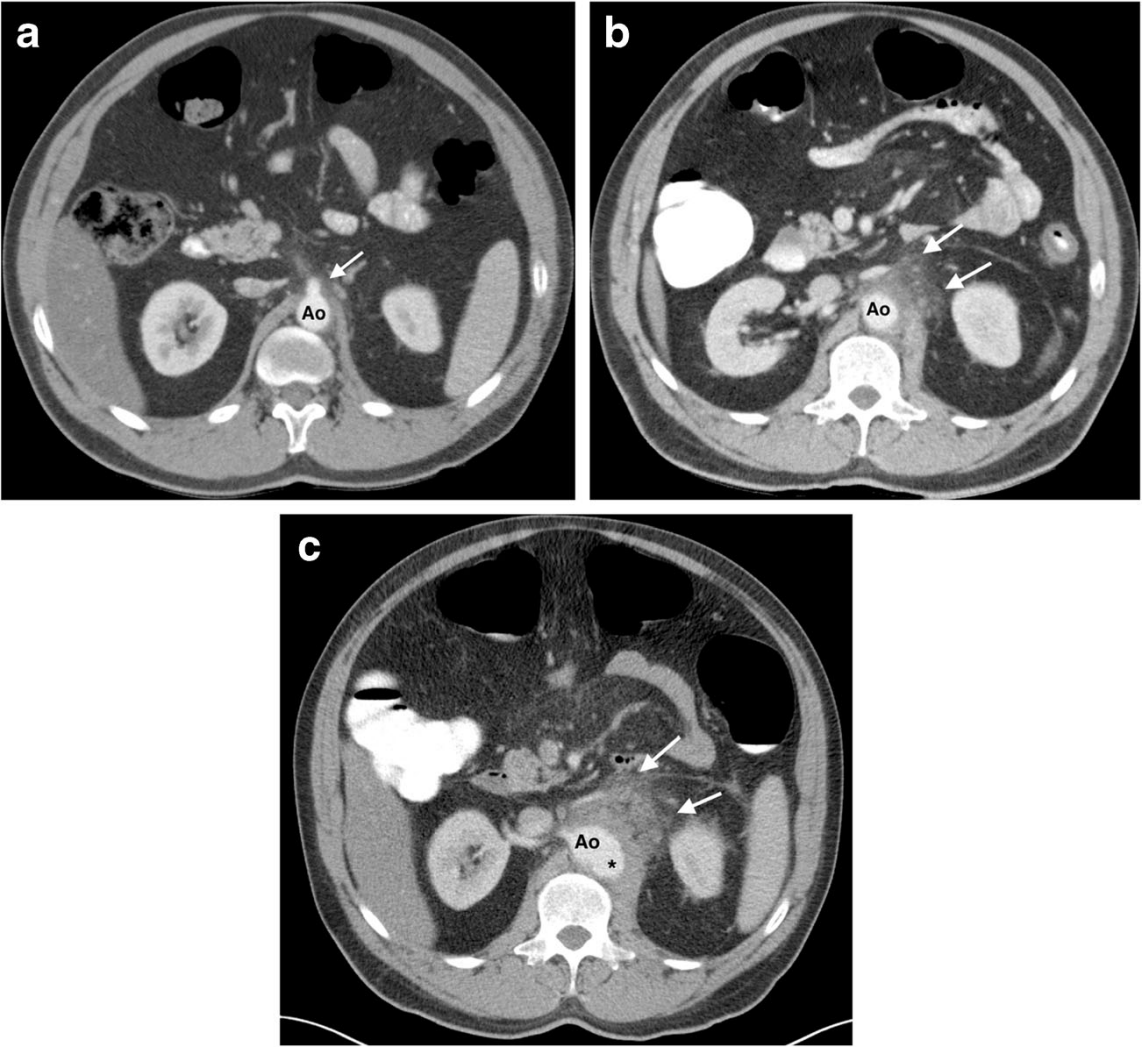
A 46-year-old man with no significant medical history presents with abdominal pain and low-grade fever. (a) An axial CT abdomen post-contrast image demonstrates ill-defined fat stranding (*arrow*) surrounding the proximal abdominal aorta at the level of the celiac artery origin. (b) A follow-up axial post-contrast CT scan of the abdomen performed 3 days later for worsening abdominal pain shows an interval increase in the severity of periaortic fat stranding (*arrows*). (c) A further follow-up axial post-contrast CT scan of the abdomen 1 week later shows the development of a saccular aortic aneurysm (*) with worsening periaortic fat stranding (*arrows*). The patient underwent surgical excision of the mycotic aneurysm with bypass grafting, and the excised aortic wall grew *S. aureus*. Despite surgical intervention, the patient died in the early postoperative period. *Ao = abdominal aorta*
